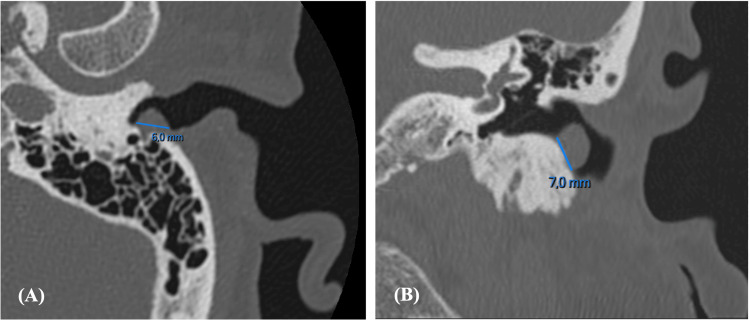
Left temporal bone CT demonstrating a pseudonodular cutaneomucosal tumor of the external auditory canal in (A) axial and (B) coronal views
